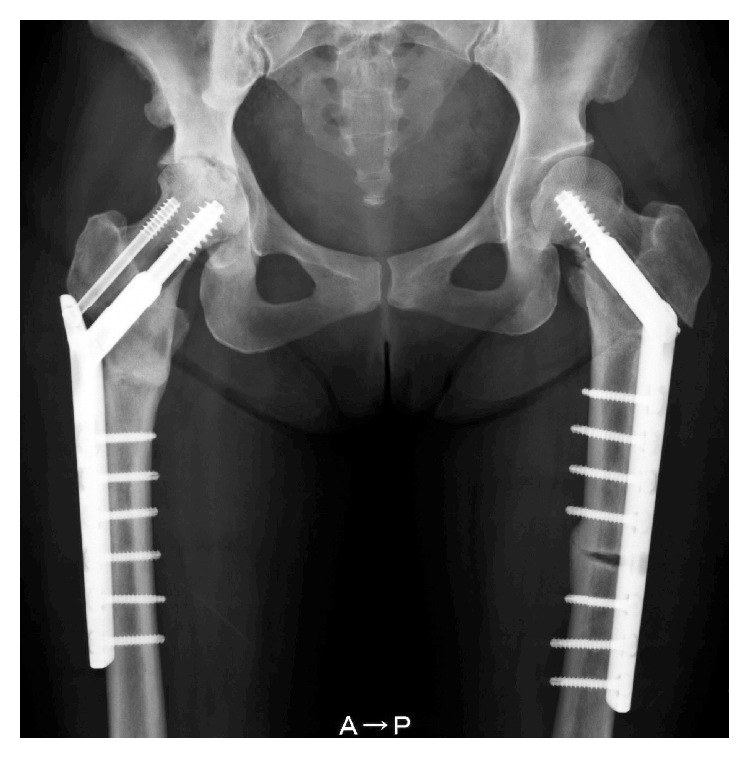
Radiograph showing advanced stage osteoarthritis of the right hip.
